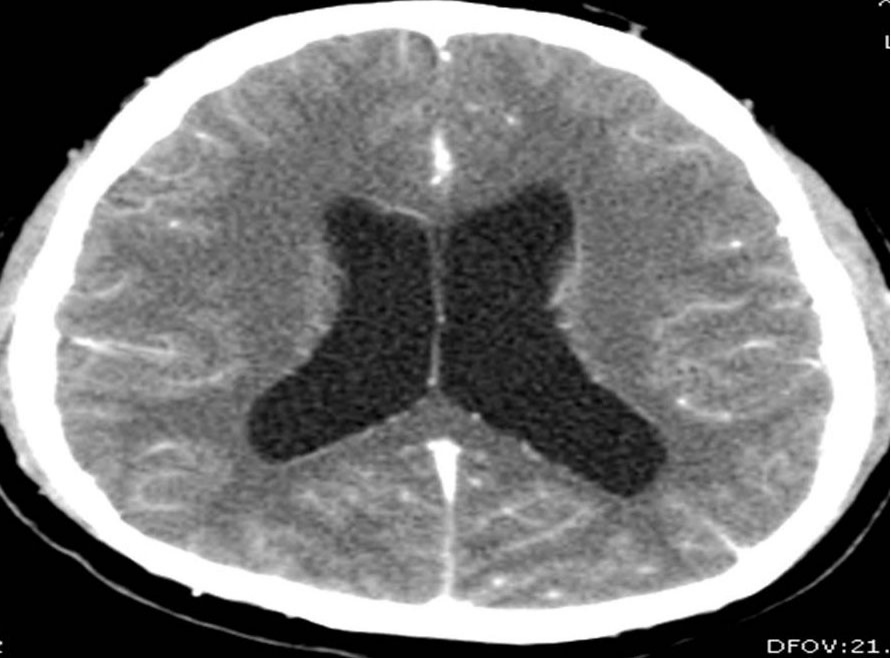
CT of his head (transverse view) showing presence of hydrocephalus and dilation of ventricles.
